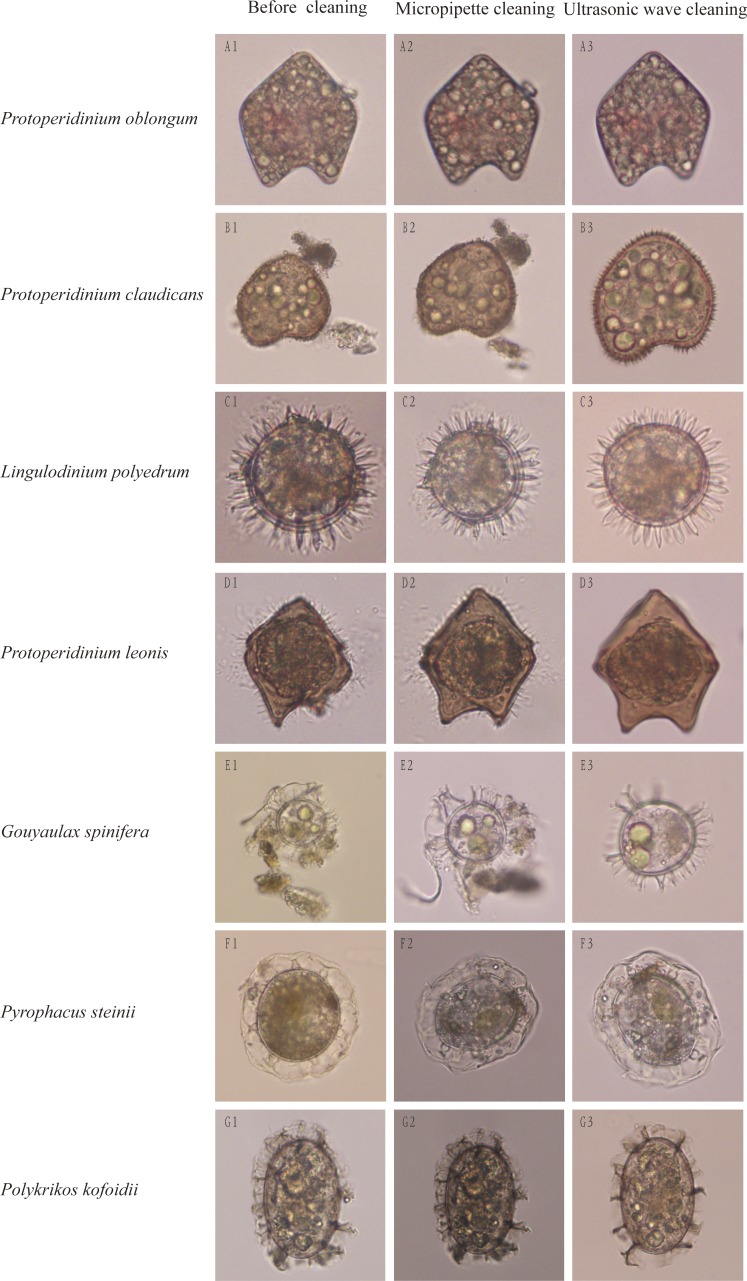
Cleaning effects on dinocysts by traditional micropipettes cleaning or our optimized ultrasonic waves-based cleaning. The left rows are dinocyst species names and the top of columns are cleaning methods.
